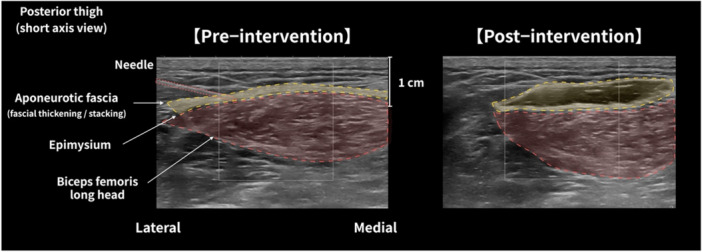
Ultrasonic short‐axis image before and after hydrorelease in the biceps femoris muscle long head. Representative short‐axis ultrasound images of the biceps femoris long head obtained before and after hydrorelease, demonstrating the anatomical relationship between the muscle and surrounding fascial layers. The yellow dashed region indicates the aponeurotic fascia exhibiting fascial thickening or stacking, identified as a hyperechoic layered structure. The red shaded region indicates the muscle belly.
